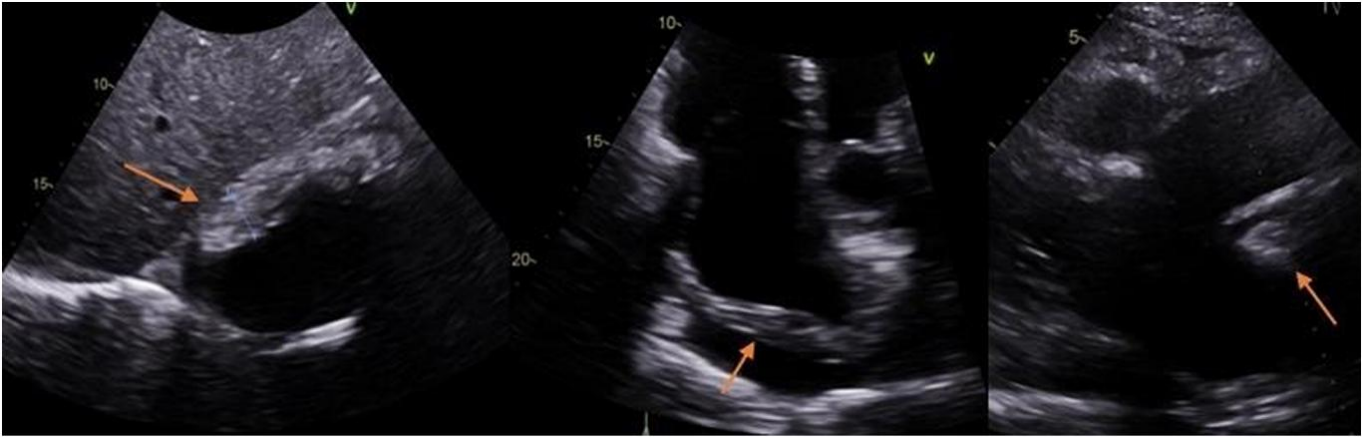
Posterior right atrial pseudotumor (subxiphoid four-chamber, apical four-chamber, and left parasternal long axis views, respectively).
